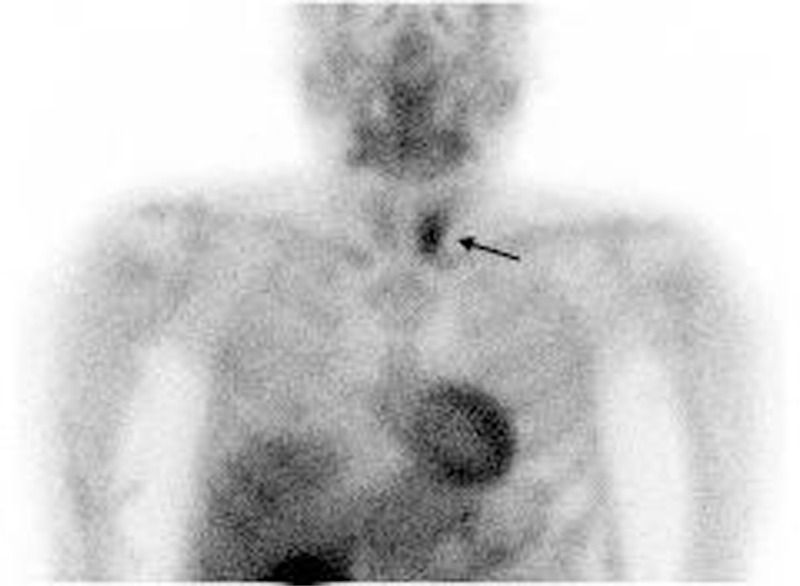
A scintigraphy showing his parathyroid adenoma (arrow).
